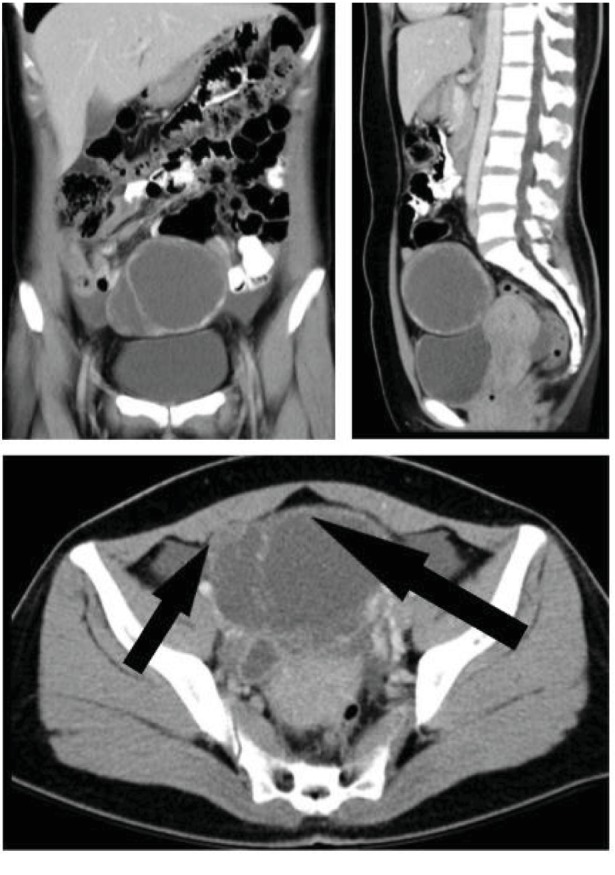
CT scan abdomen/pelvis shows a left adnexal, complex cystic lesion with enhancing, solid nodules along its wall (black arrows), likely arising from the left ovary.
